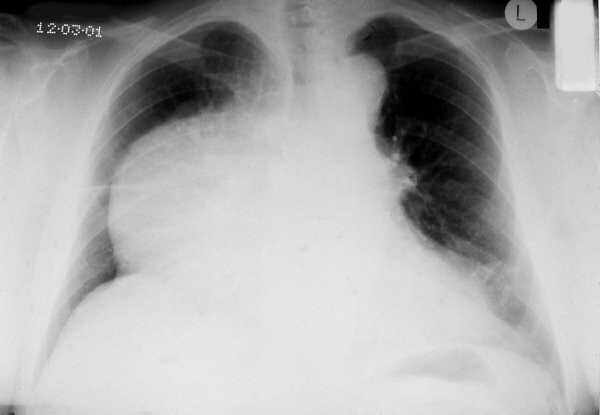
The chest x-ray, showing a large mass projecting over the right lung field.
